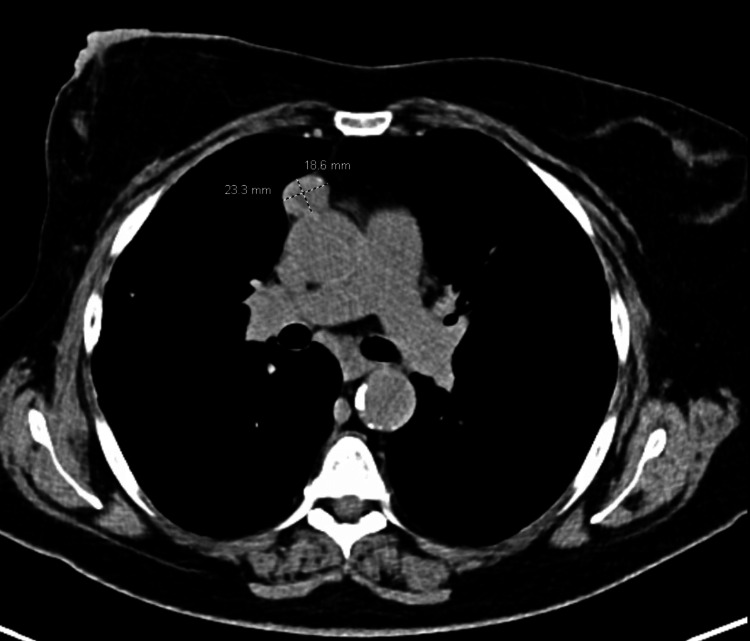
CT chest showing prevascular mass anterior to the ascending aorta measuring 1.9 X 2.3 cm
